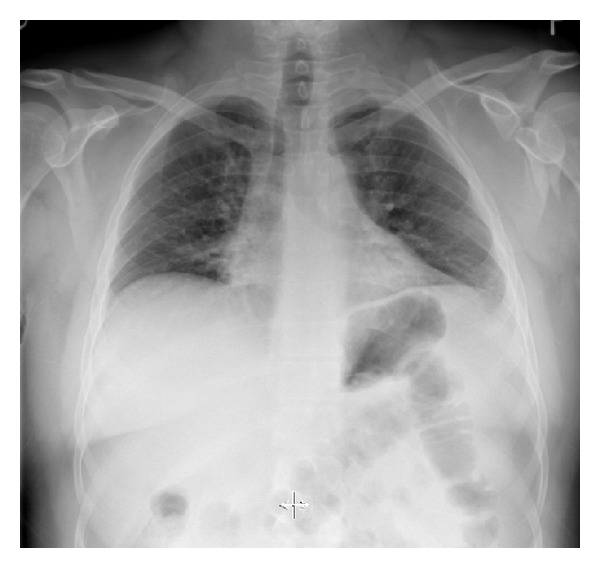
Anteroposterior chest radiograph demonstrating a left scapular fracture.
